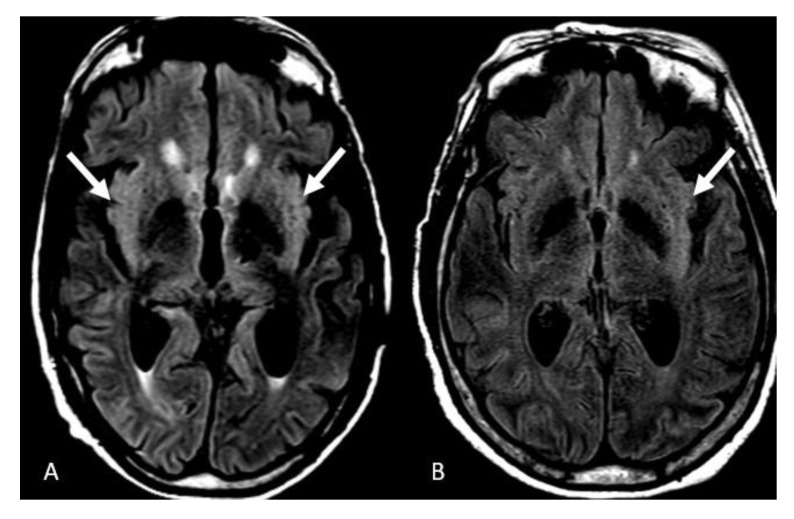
Insular involvement. (A) Axial FLAIR images demonstrate bilateral FLAIR hyperintensities in the insular cortex. (B) isolated left insular involvement. Image has been windowed manually to improve the visibility of signal abnormality.
